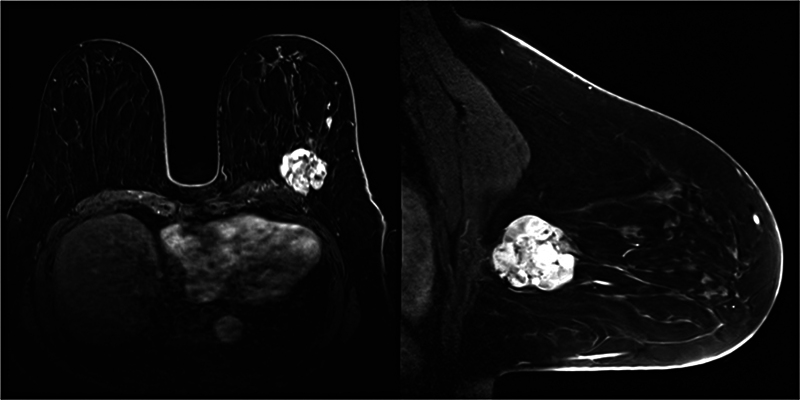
Preoperative contrast-enhanced breast MRI. A 43-mm lobulated tumor was identified in the upper outer quadrant of the left breast. The contrast-enhanced pattern displayed a fast-washout pattern with cystic structures observed within the tumor.
